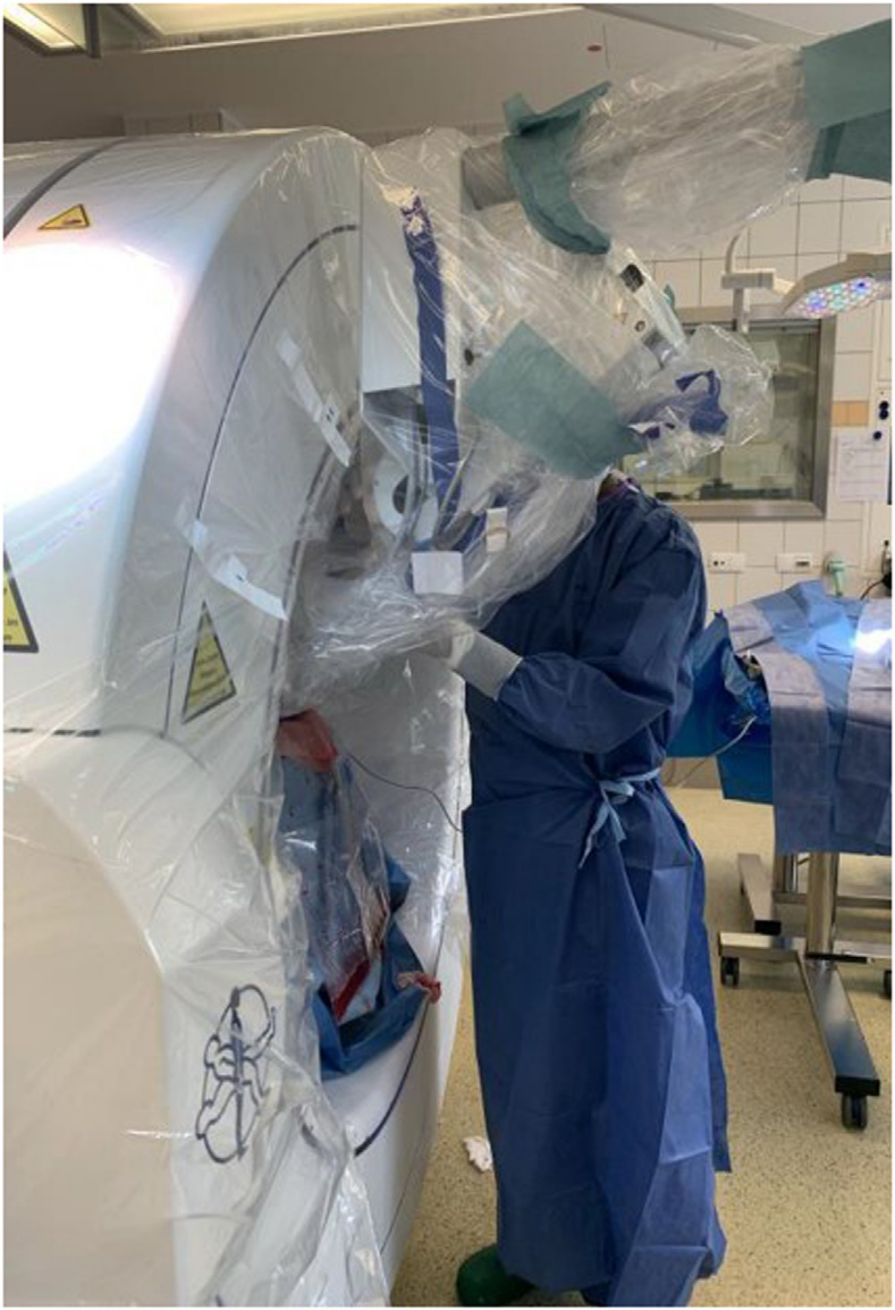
Simultaneous coupling of the in-room cone beam computed tomography kilovoltage intraoperative radiation therapy device.
